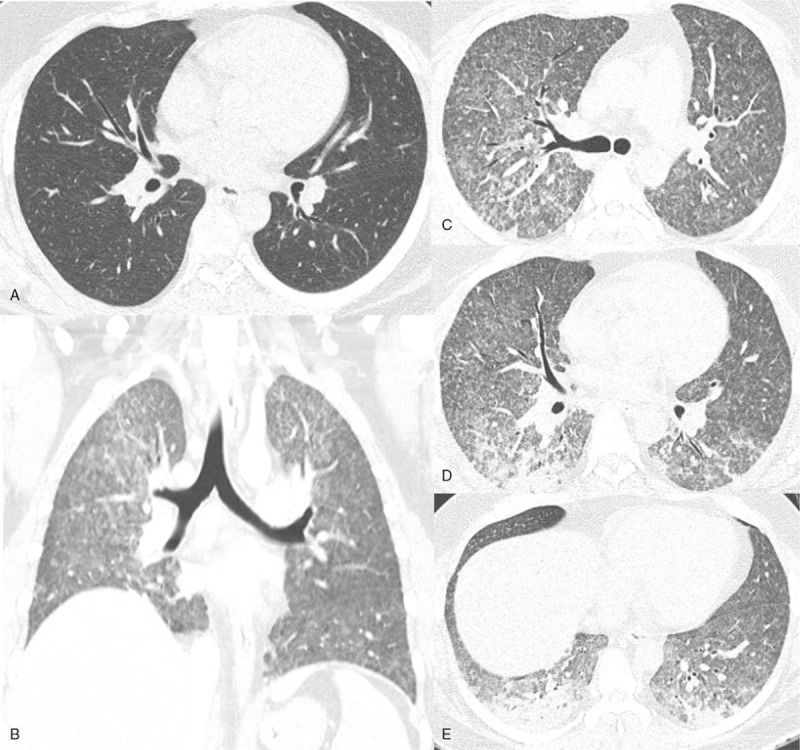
(A) Chest HRCT scanned initially upon his visit to the emergency department, without any pathological findings. (B-E) Chest HRCT scanned 2 weeks after initiation of corticosteroid therapy, when a rapid deterioration of clinical and radiological conditions occurred. (B, C, and D) Axial and (E) coronal images of HRCT show diffuse distribution of indistinct micronodules in all lobes of the lung. Notice there is no subpleural sparing. Also, there is non-homogeneous distribution of ground-glass opacities and dense consolidation in dependent regions, typically featured in ARDS.
